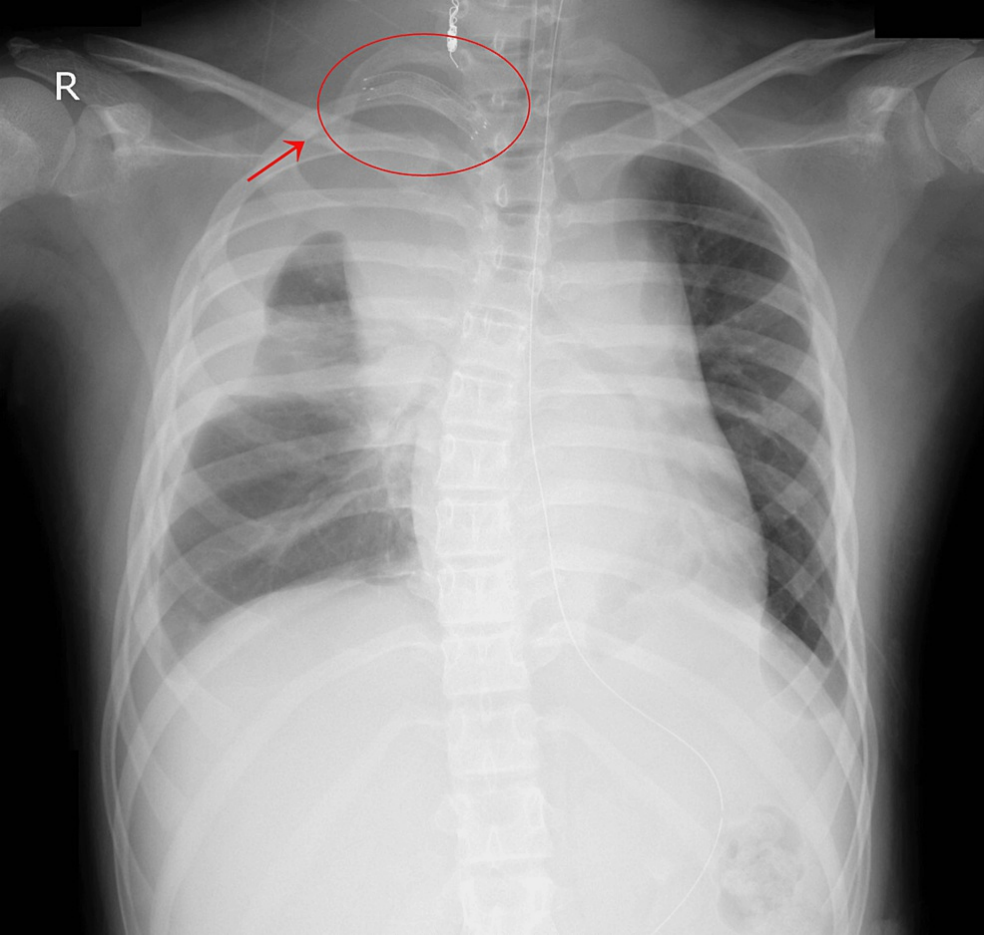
Chest X-ray after stenting on day 0 Chest X-ray showing the stent placed in the right subclavian artery (red arrow and red circle) on day 0.
